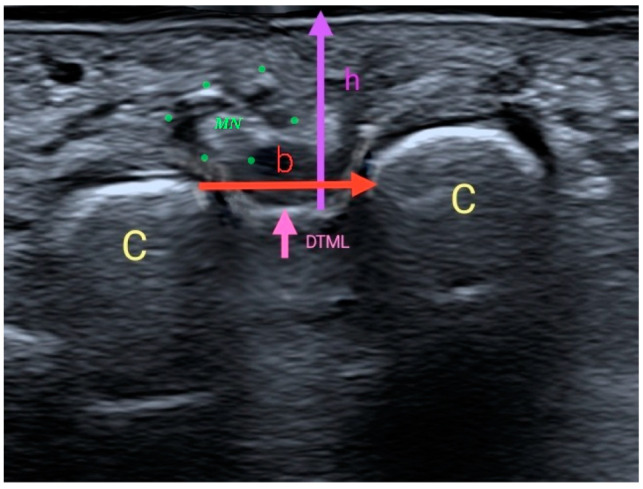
Ultrasound imaging of the measurements.C3 and C4: metatarsal heads 3 and 4 (M3 and M4); DTML: deep transverse metatarsal ligament; h: height: distance between the DTML and the plantar skin in the middle area between M3 and M4; b: base: distance between M3 and M4; MN: Morton’s neuroma.
